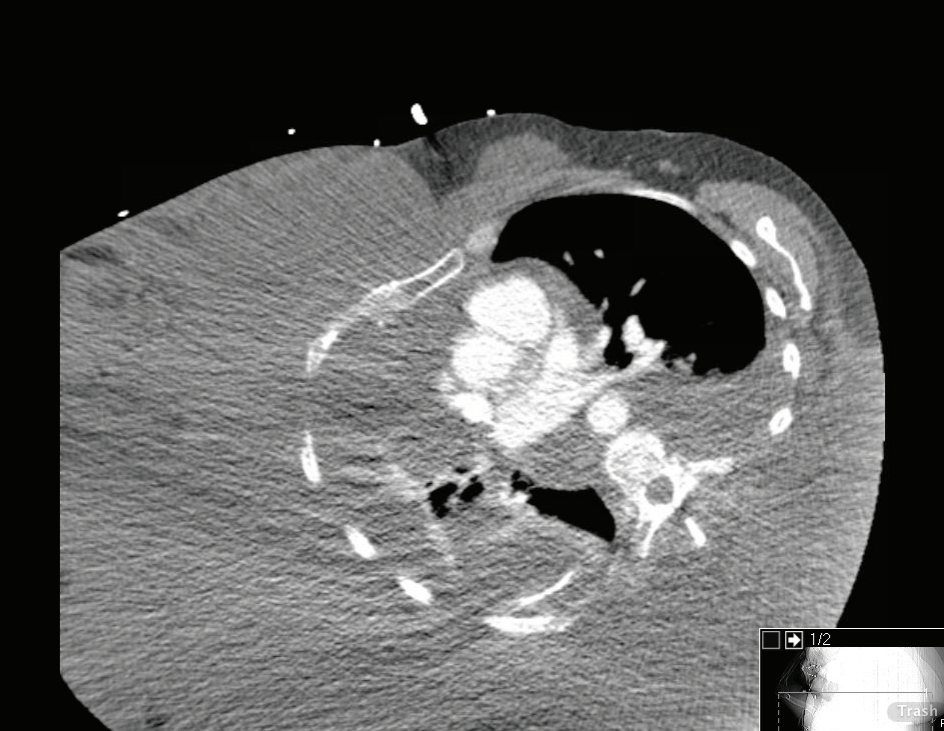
Axial CT chest demonstrating swelling of right hemithorax.
